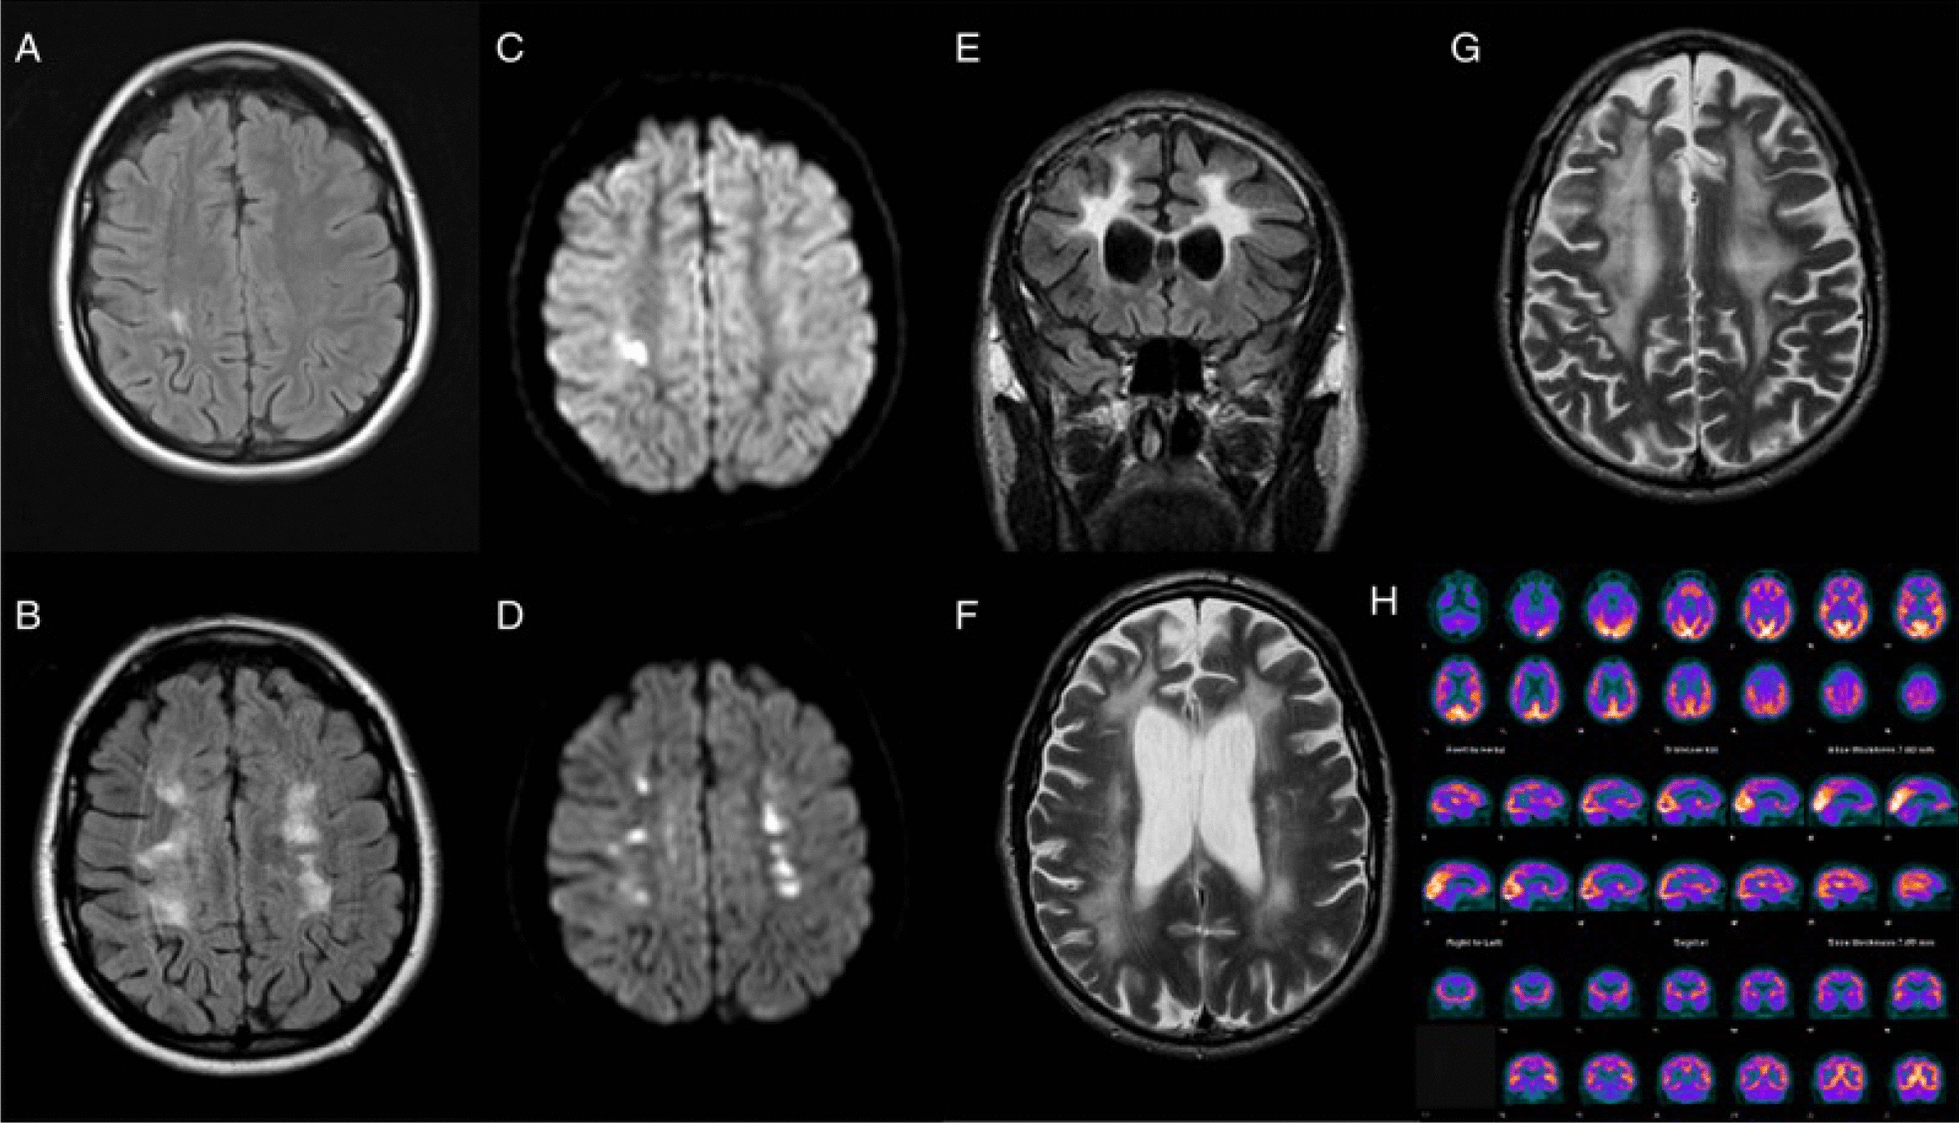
Serial FLAIR/DWI (A–E), T2- T2-weighted imaging (F-G), and FDG-positron emission tomography (H) demonstrating changes as seen in neurodegenerative AHS; FDG: Fluorodeoxyglucose [53]
